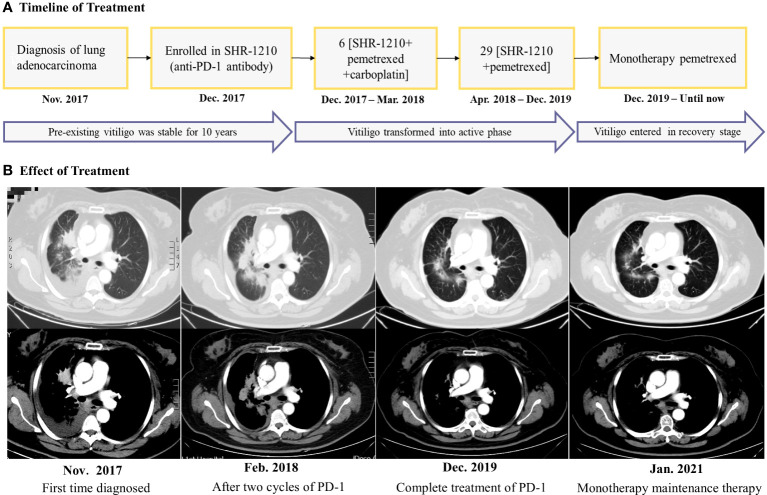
(A) Timeline for the diagnosis, treatment, and changes of the vitiligo condition. (B) Chest computed tomography scans revealed the clinical response during the treatment. The efficacy evaluation was stable disease after the two cycles of ‘SHR-1210 combined with pemetrexed and carboplatin, but as the treatment time was prolonged, the tumor density gradually decreased, suggesting that the tumor has been effectively controlled.
